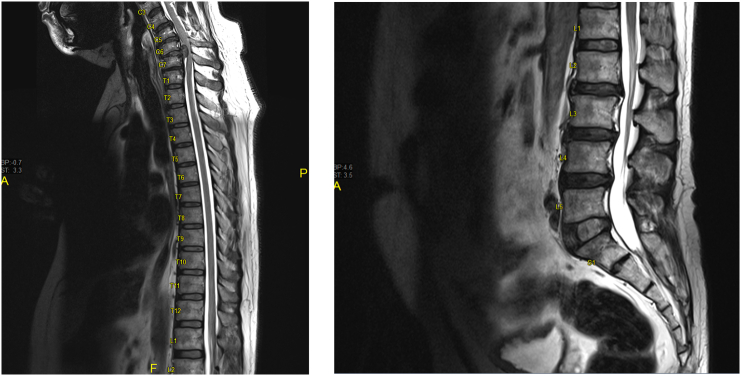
MRI thoracic and lumbar spine (lateral view) showing no major disc herniation or nerve root impingement.
